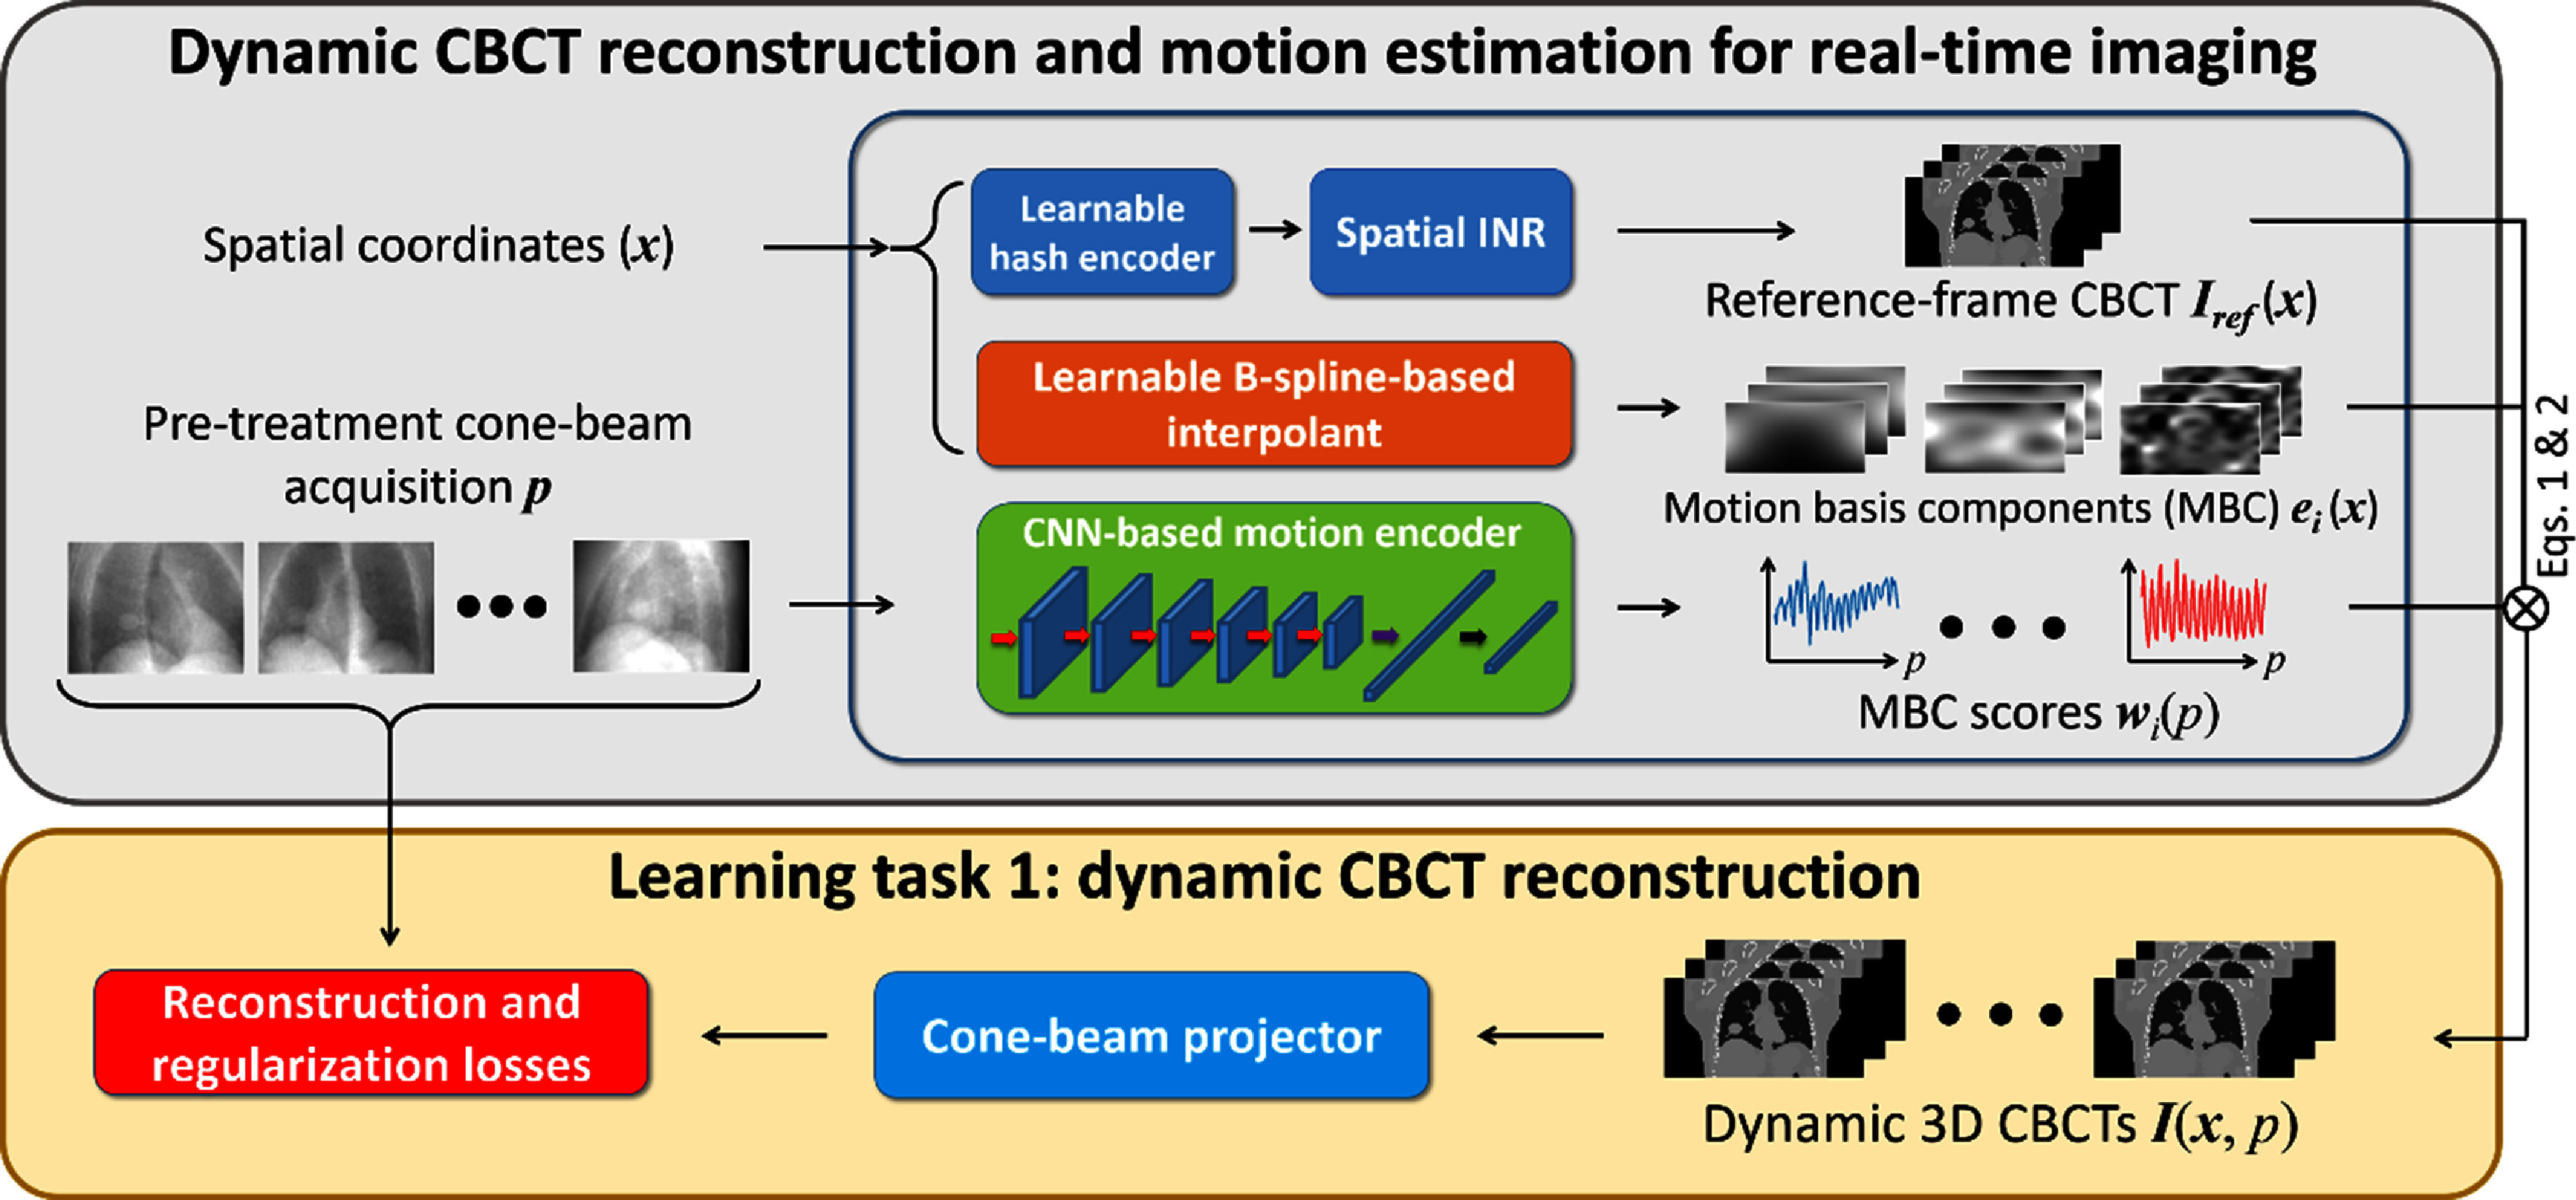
Learning task 1: dynamic CBCT reconstruction. The learning task of dynamic CBCT reconstruction is driven by maximizing the similarity between the digitally reconstructed radiographs (DRRs) of the motion-resolved CBCTs and the corresponding cone-beam projections. To regularize the ill-posed spatiotemporal inverse problem, regularization losses are incorporated into the training objectives.
